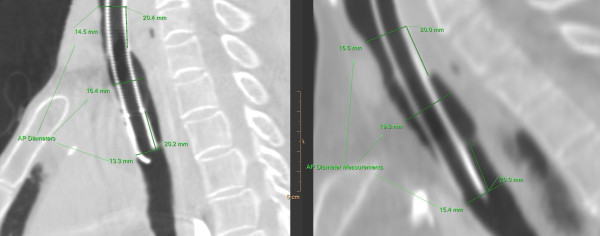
Examples of the CT images in a sagittal plane of the LVLP (left) and HVLP cuff (right) *in situ*, delineating the mid cuff level, 20 mm above and 20 mm below cuff measurements.
